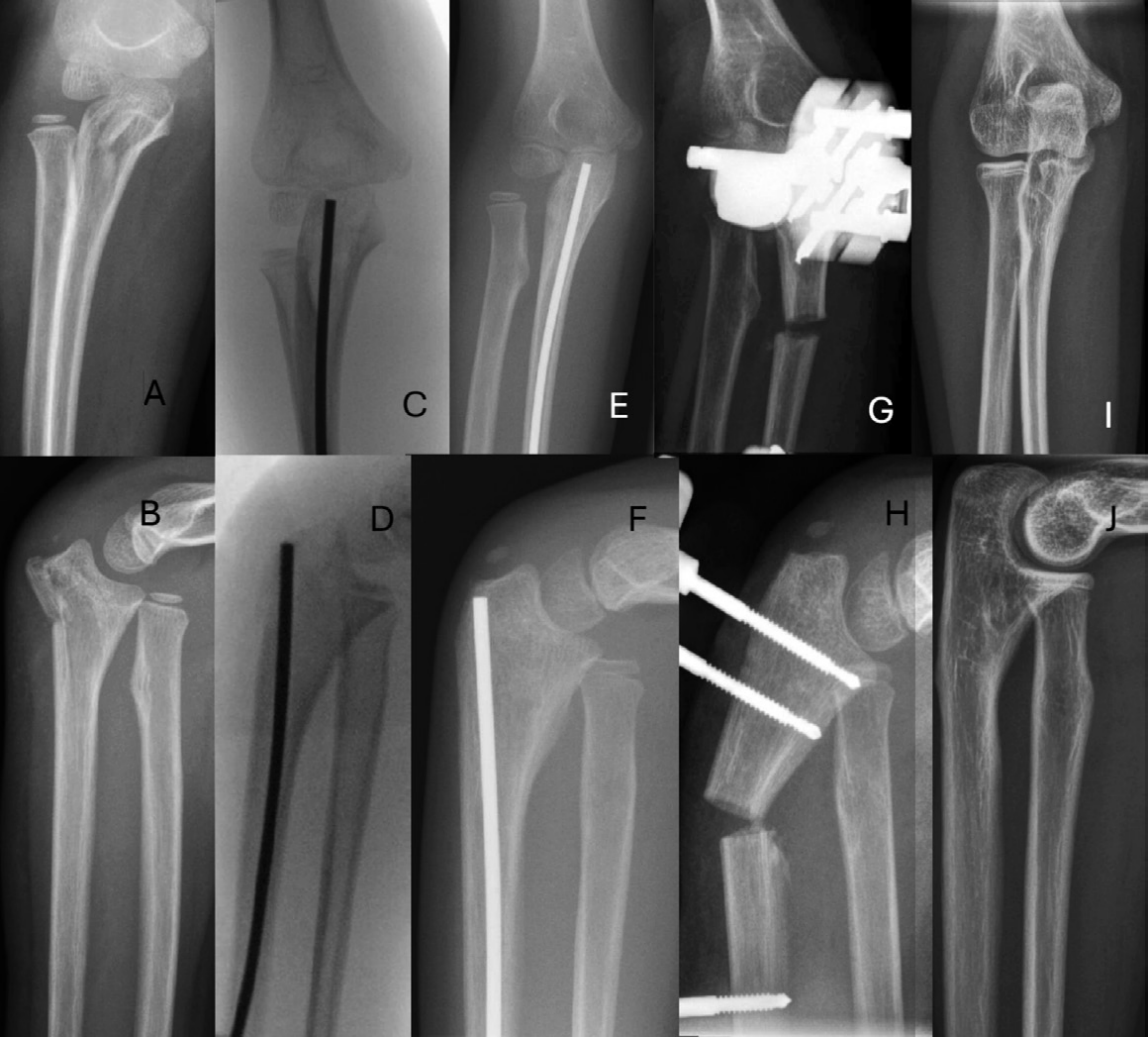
A 5-year-old girl with a Bado type III Monteggia fracture (A, B) treated with closed reduction of the radiohumeral joint (RHJ) and flexible intramedullary nailing of the proximal ulna fracture (C, D). 3 months from surgery persistent lateral dislocation of the RHJ was observed (E, F) and an ulnar osteotomy with an open reduction of the RHJ was performed as described by Hasler et al. [13] (G, H). Radiographs 6 years later show a congruent RHJ and normal alignment of the ulna (I, J).
